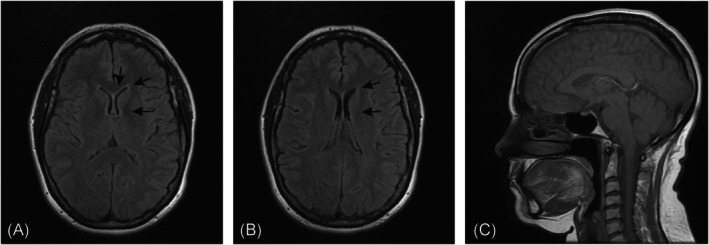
Magnetic resonance tomography of the brain at 48 years of age. (A and B) Bilateral symmetric discrete and diffuse enhanced signal in periventricular white matter (arrows) (T2/FLAIR). (C) Sulci appear normal and no atrophy is seen (T1).
